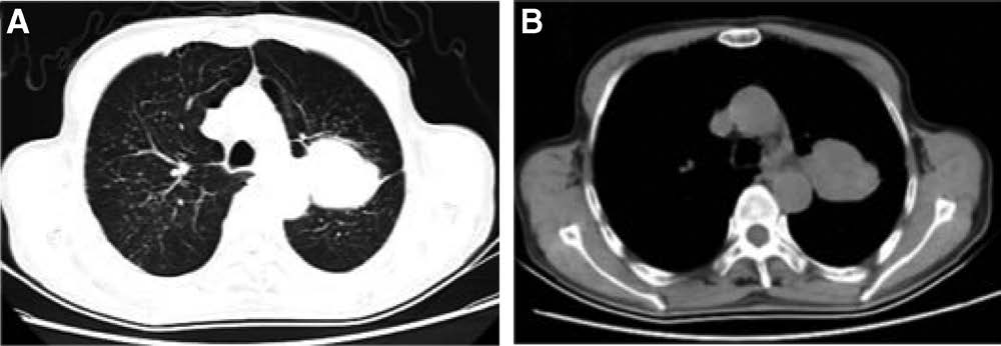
(A and B). Chest CT revealed an irregular mass in the upper lobe of the left lung. CT = computed tomography.
